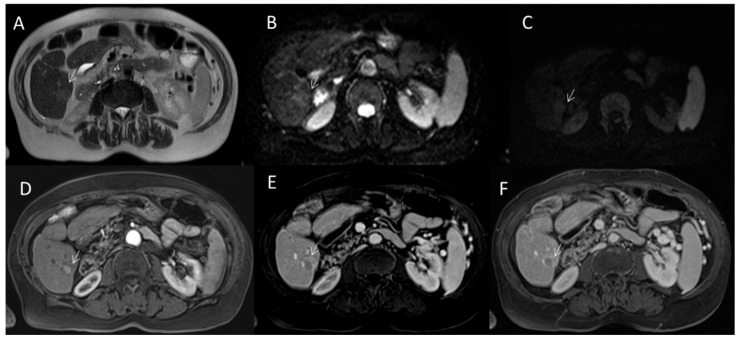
HCC on VI seg. MRI assessment. The lesion (arrow) shows hyperintense signal on T2-W sequence (A), restricted diffusion on DWI sequences ((B): b50 s/mm^2^ and (C): b800 s/mm^2^) with APHE during arterial phase (D) of contrast study, with washout and capsule appearance during portal (E) and late phase (F) of contrast study.
